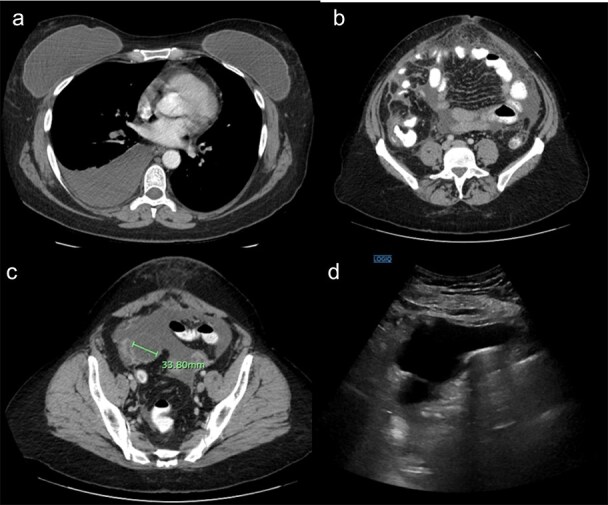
(a) Demonstrating right pleural effusion, likely from pulmonary coccidioidomycosis. (b) Demonstrating thickened anterior omental fat concerning for peritoneal carcinomatosis. (c) Demonstrating a right adnexal mass. (d) Ultrasound demonstrating a large amount of ascites.
